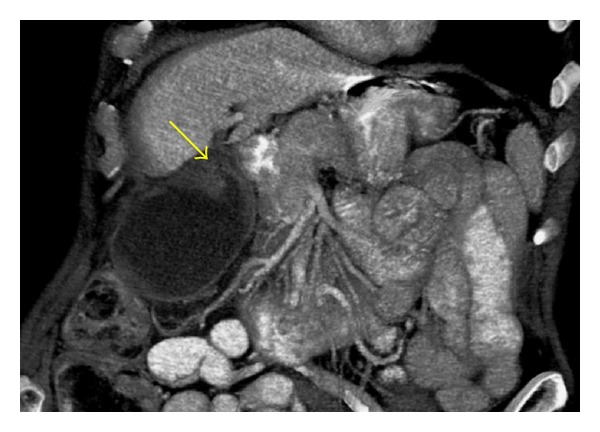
3-Dimensional volume reconstruction CT abdomen to confirm a distended gallbladder with a clear transition point (arrow), pericholecystic fluid, inflammatory stranding and enhancement of the gallbladder wall.
